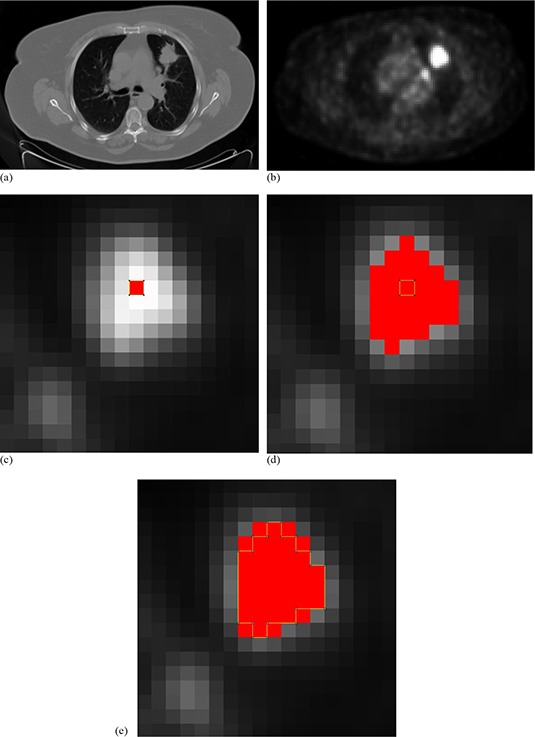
CT image (from FDG PET/CT exam) for lesion #62 (a). FDG PET image (Bmin=464) (b). Zoom on FDG PET image, maximum intensity in the lesion appears in red (Tmean=Tmax=22146), first iteration (c) — FDG lesion uptake in red (%Tmean=52.88% and Threshold=11711, that gives Tmean=17385); second iteration (d) — FDG lesion uptake in red (%Tmean=55.92% and Threshold=9722, that gives Tmean=16214). Convergence at the third iteration (e) (%Tmean=56.83% and Threshold=9215) to a constant lesion area on the PET image (Area $\text{PET}=62{\text{mm}}^{2}$).
